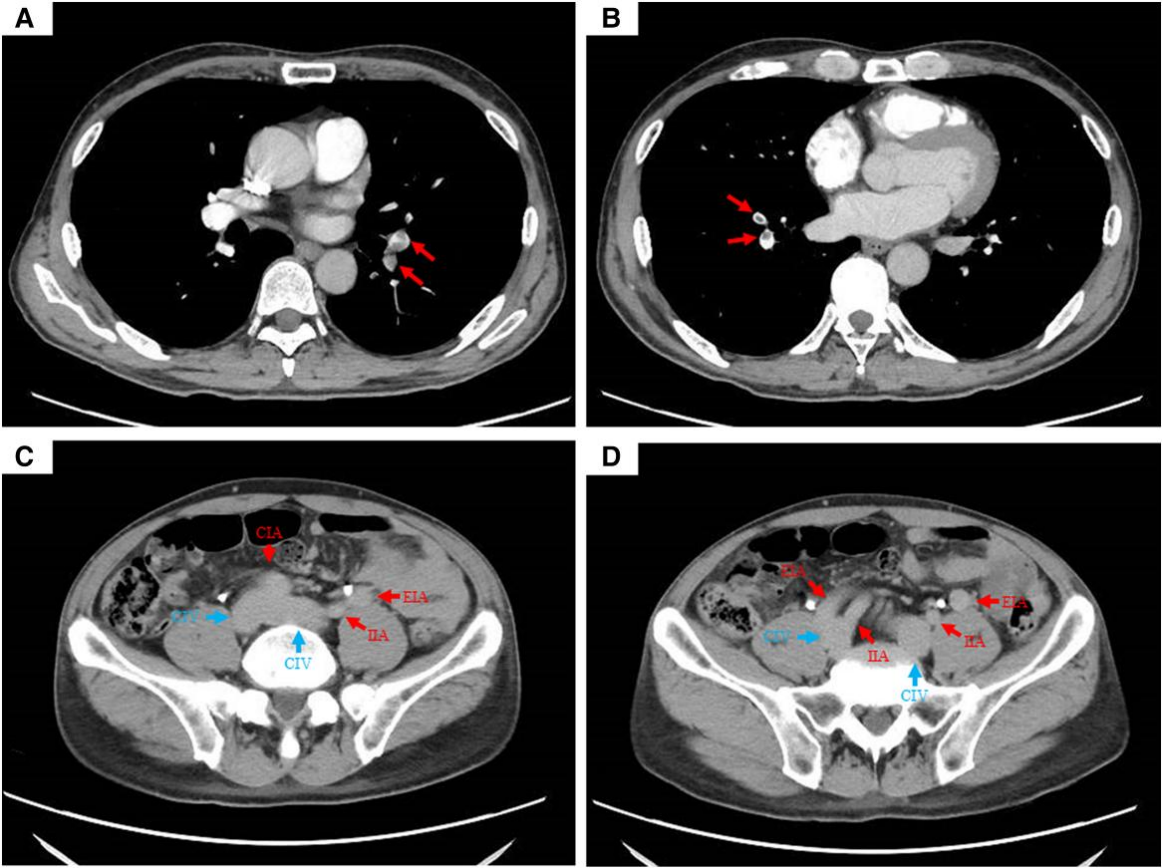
Contrast-enhanced computed tomography. (*A*, *B*) Multiple thrombi in both pulmonary arteries (arrow). (*C*, *D*) No compression of the left common iliac vein was observed. The right external iliac vein ran between the right internal iliac artery and external iliac artery but could not be adequately evaluated. CIA, common iliac artery; CIV, common iliac vein; EIV, external iliac vein; IIA, internal iliac artery; EIA, external iliac artery.
